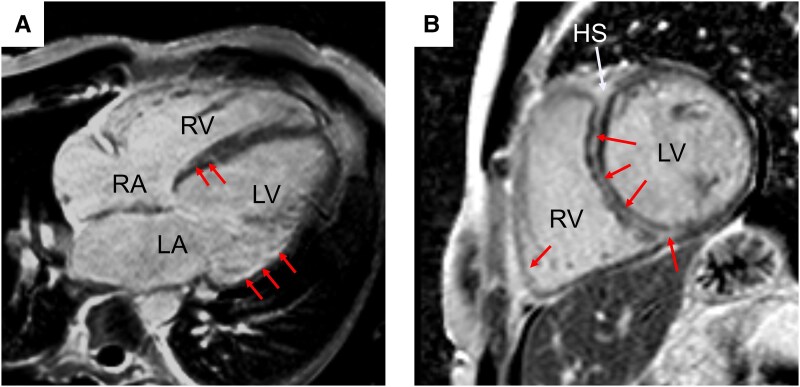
Cardiac magnetic resonance imaging eight months after symptom onset. The eight-month follow-up cardiac magnetic resonance imaging showed persistent but overall reduced late gadolinium enhancement (arrows) in phase-sensitive inversion recovery imaging. HS, hook sign; LA, left atrium; LV, left ventricle; RA, right atrium; RV, right ventricle.
